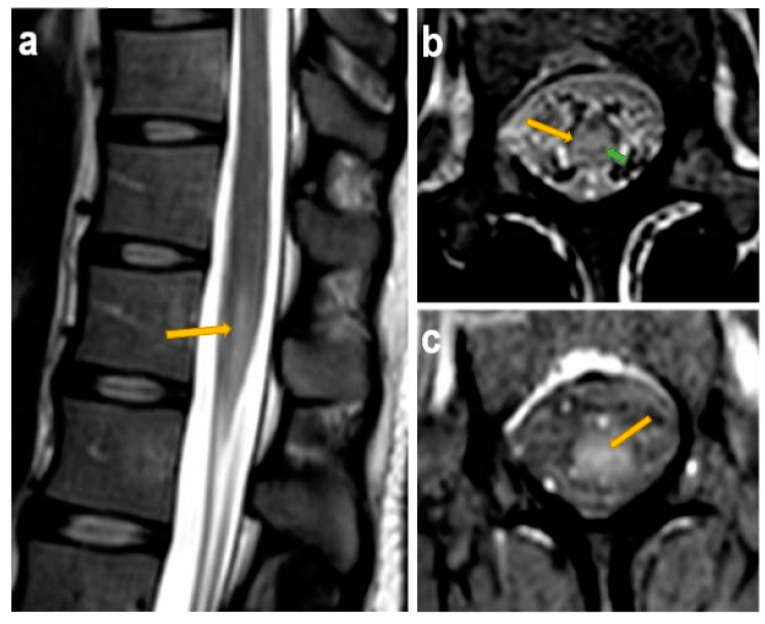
Magnetic resonance imaging of the lumbosacral spine. Sagittal (a) and axial (b) T2 weighted showing enhancement (yellow arrows), and axial T1-weighted sequence (c), showing contrast enhancement of the medullary cone. Panel (b) also displays sparing of some nerve bundles (green arrow).
